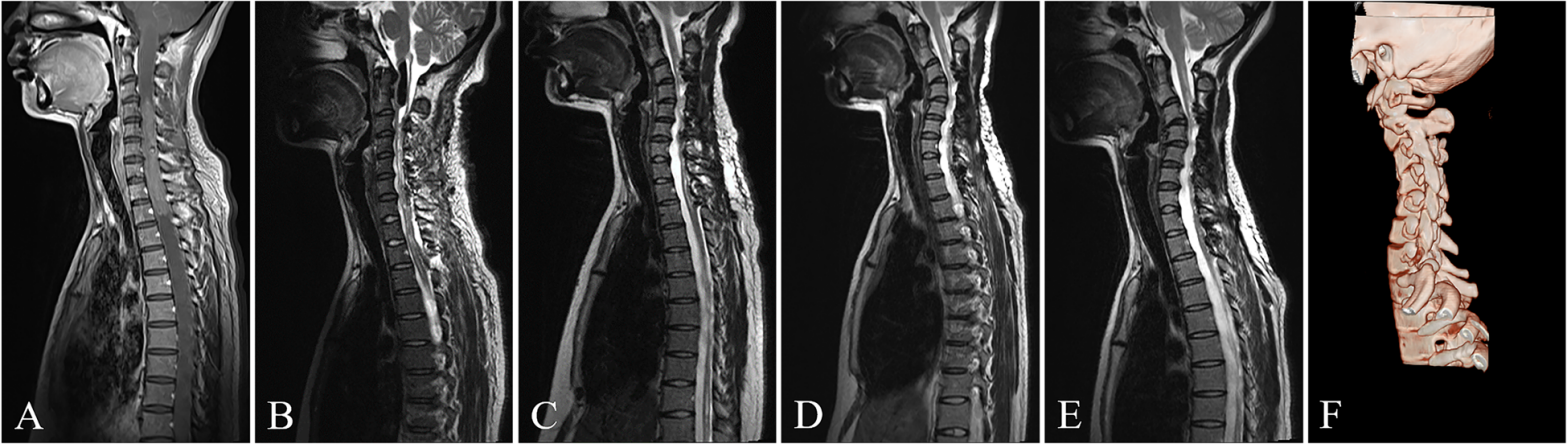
The T2-weighted MR images shown was a 30-year-old woman who underwent resection of the ependymoma spanning from C3 to T2. a Preoperative MR images showed a huge tumor and the cervical spine had lost its normal curvature. b Postoperative MR images during postoperative hospitalization showed complete resection of spinal cord tumor and almost no change in cervical curvature. c The follow-up MR image showed progressive cervical kyphosis 6 months after the operation. d, e The follow-up MR image showed deterioration of progressive cervical kyphosis 13 months and 24 months after the operation, respectively. f The CT three-dimensional reconstruction image showed the cervical kyphosis 24 months after the operation. At that time, the patient complained that her left upper limb was numb
